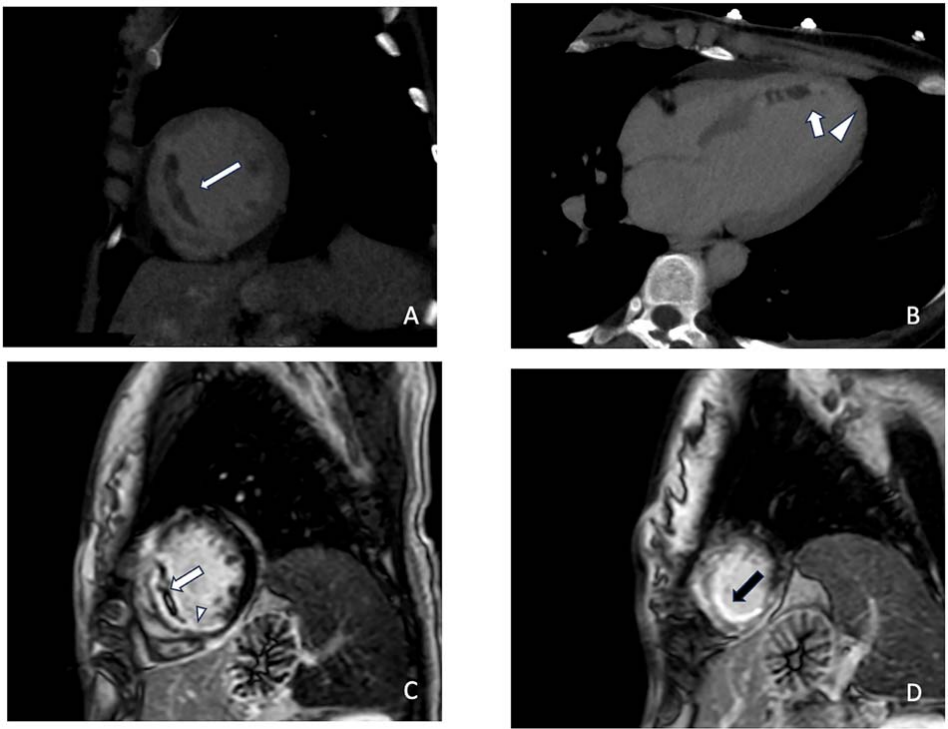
June 2023 LIE-CT scan on short-axis (A) and four-chamber (B) reconstructions show areas of LIE in the mid-septal (white arrow in A and B) and apical segment (white arrowhead in B). Note the hypodensity area included in the LIE of the septal segment compatible with MVO. One week later, CMR confirmed superimposable extension of the infarcted areas with LGE clearly visible on short-axis (C, white arrow) and long-axis four-chamber (D, black arrow). MVO: microvascular obstruction.
